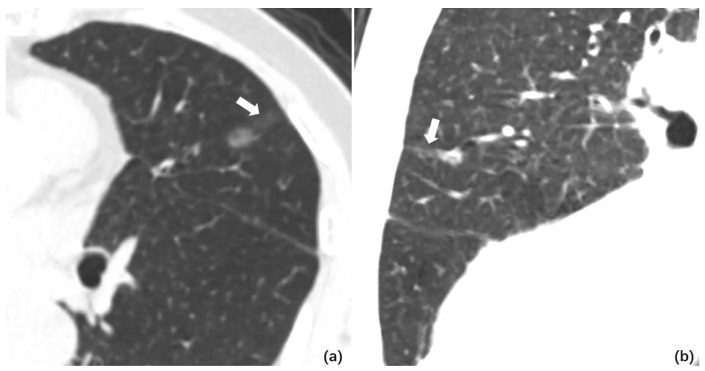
Example of Ground Glass Ribbon on CT Imaging. (a) Axial cut of preoperative CT imaging of a lung nodule (post-resection confirmed STAS+) with the ground glass ribbon sign: a nodule with blurred line (or “ribbon”) that arises from the nodule edge and extends to the surrounding lung (white arrow). (b) Multiplane reconstruction from same CT imaging from (a) with improved visualization of the ground glass ribbon sign (white arrow). Reproduced via Creative Commons CC BY license without alteration from: Qi L, Xue K, Cai Y et al. Predictors of CT Morphologic Features to Identify Spread Through Air Spaces Preoperatively in Small-Sized Lung Adenocarcinoma. *Font Oncol*. 2020;10:548430. Doi: 10.3389/fonc.2020.548430 [89].
